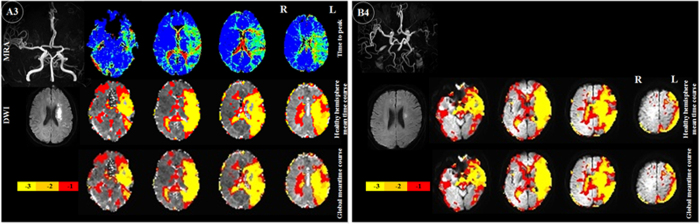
Perfusion injury in subacute stroke patients with left middle cerebral artery (MCA) severe stenosis/occlusion. A3, Comparison of time delay maps from global mean time course (bottom row), healthy hemisphere mean time course (middle row) and time to peak (TTP, upper row). TTP and TSA demonstrated comparable hypoperfusion areas. A3&B4, the time delay areas acquired based on global and healthy hemisphere mean time course were sizable. MRA = magnetic resonance angiography, DWI = diffusion weighted imaging. −1, −2, −3 in color bar indicate −1TR, −2TR, −3 TR time shift.
